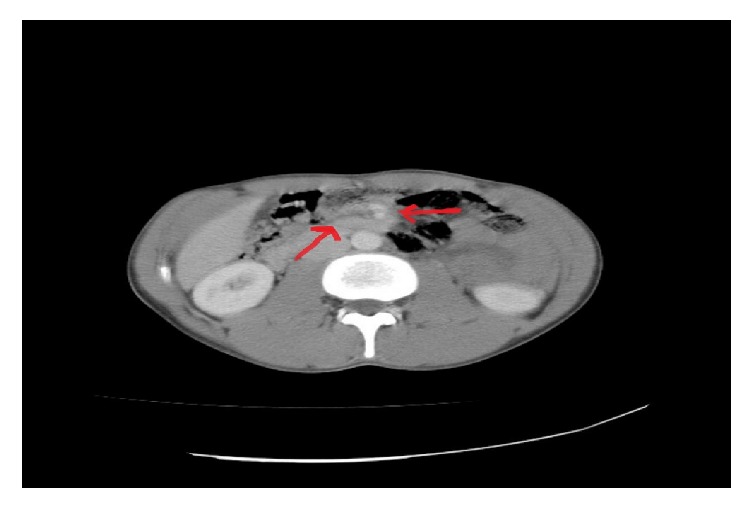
Axial contrast enhanced CT showing characteristic whirlpool sign. The structure between the two arrows shows whirlpool sign.
